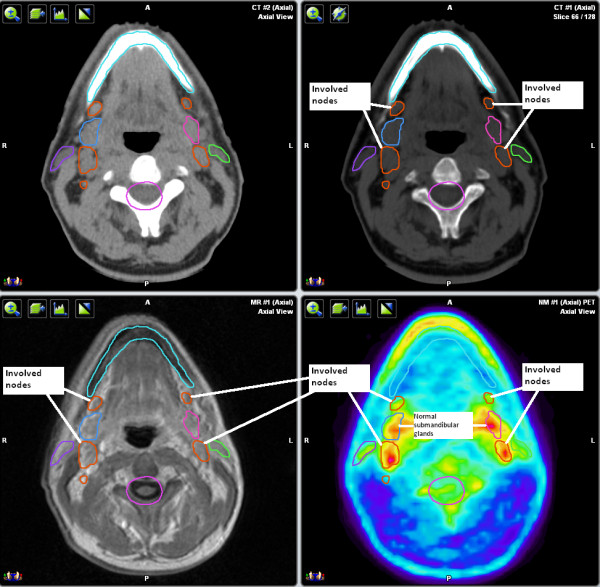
CT, MRI, and PET axial views. These enlarged lymph nodes were shown to be FDG-PET-avid, changing the stage from IAE to IIAE.
